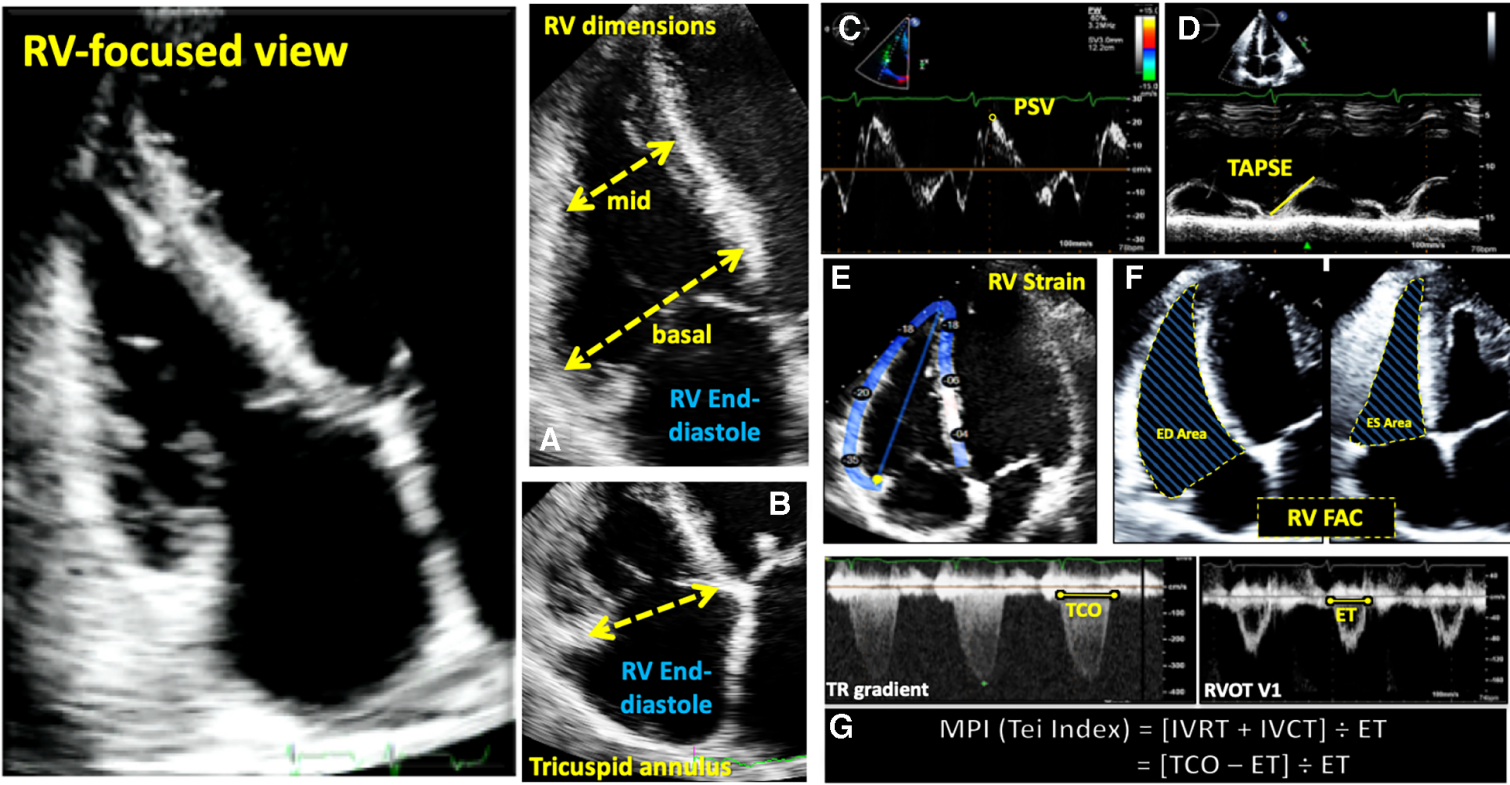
Guideline-recommended right ventricular size and function assessment. All measurements are performed in the RV focused apical 4-chamber view (far left panel). (A) RV mid-ventricular and basal dimensions obtained at end-diastole in the RV-focused view; (B) tricuspid annulus diameter obtained at end-diastole starting at the hinge-point of the non-septal TV leaflet and ending at hinge-point of the septal TV leaflet; (C) depiction of doppler-derived tricuspid lateral annular systolic velocity (S’) or peak systolic velocity (PSV); (D) m-mode is used to measure longitudinal displacement of the lateral tricuspid annulus in systole to yield tricuspid annular systolic excursion (TAPSE); (E) region of interest used to guide free-wall and global RV strain assessment; (F) RV areas obtained at end-diastole and end-systole to compute fractional area change; (G) Calculation of the myocardial performance index (MPI) defined as the sum of isovolumic contraction time the (IVCT) and isovolumic relaxation time (IVRT) divided by ejection time (ET) obtained from the right ventricular outflow tract initial velocity (RVOT V1). The sum of IVCT and IVRT is equal to the difference between the interval from cessation to onset of the tricuspid inflow (TCO) and ET.
